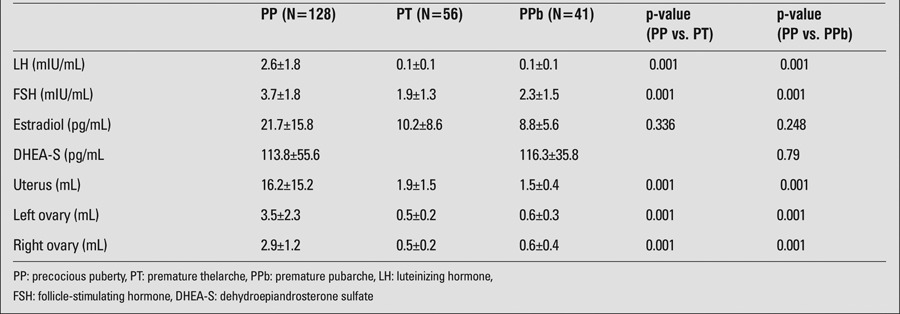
Hormonal levels and ultrasonographic evaluation in PP, PT, and PPb groups
